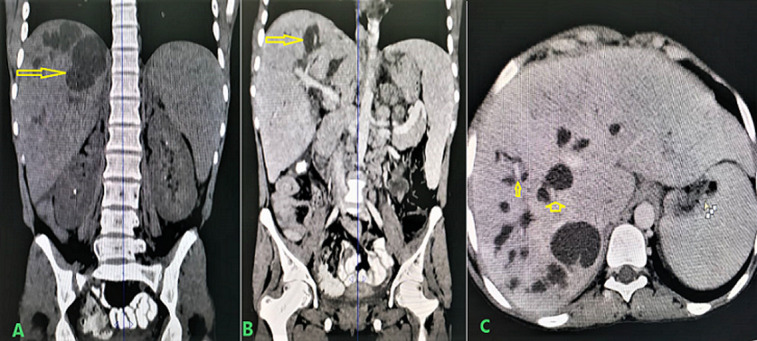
abdominal CT scan showing intrahepatic cystic dilatation (A); non-contract CT scan in coronal views (B); contract CT scan in coronal views (C); contract CT scan in axial views
